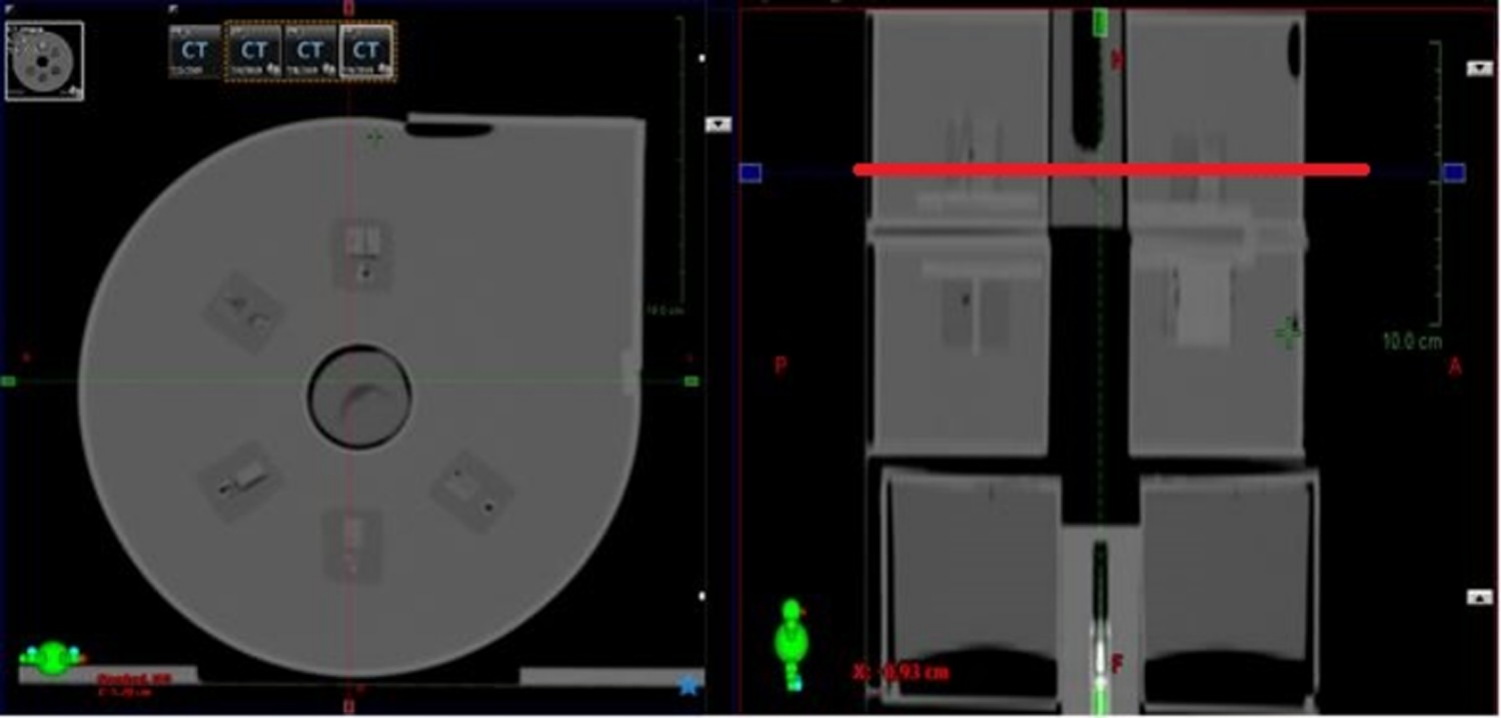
CT image of the high-spatial-resolution slab.
